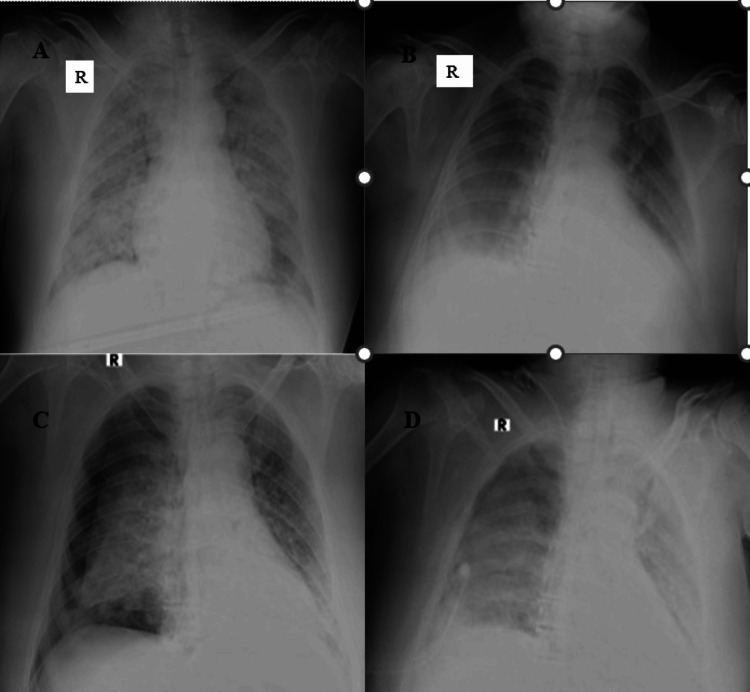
Figure 4A: Chest supine radiograph (anteroposterior (AP) view) of a 64-year-old male patient showed multiple nodular opacities, ill-defined ground glass opacities, and patchy consolidation areas in bilateral lung parenchyma involving > 75% of parenchyma. Radiographic disease severity score was eight (severe grade). Figure 4B: Follow-up chest AP supine radiograph of the same patient on day 10 with mechanical ventilation revealed diffuse areas of GGOs in the bilateral mid and lower lung zones with peripheral and basal high-density areas, suggestive of consolidation. The lung involvement was 25% to 50% bilaterally, and score four represented radiographic improvement. Figure 4C: Follow-up chest AP supine radiograph of the same patient on day 12 showed the development of pneumothorax on the right side (possibly barotrauma-related). Figure 4D: Follow-up chest AP supine radiograph of the same patient on day 14 revealed re-expansion of the right lung with minimal residual pneumothorax. Diffuse ground glass opacities were seen in the entire right lung field, and diffuse areas of consolidation were seen in the entire left lung field.
